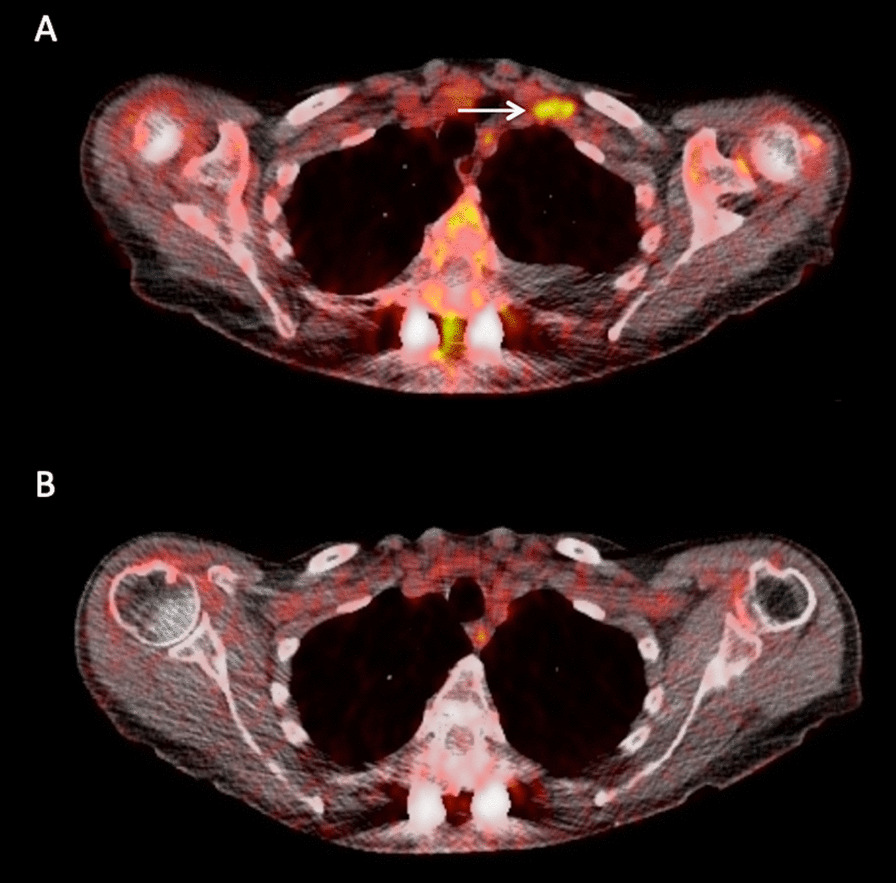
Transaxial PET/CT images at the thoracic level of a 69-year-old female MM patient before and after therapy. A Baseline PET/CT demonstrated a hypermetabolic, intramuscular, soft-tissue lesion in the thoracic wall with no relationship to the bone (arrow) as a sign of EMD. B Follow-up PET/CT showed a complete metabolic remission of the EMD as response to treatment (B)
